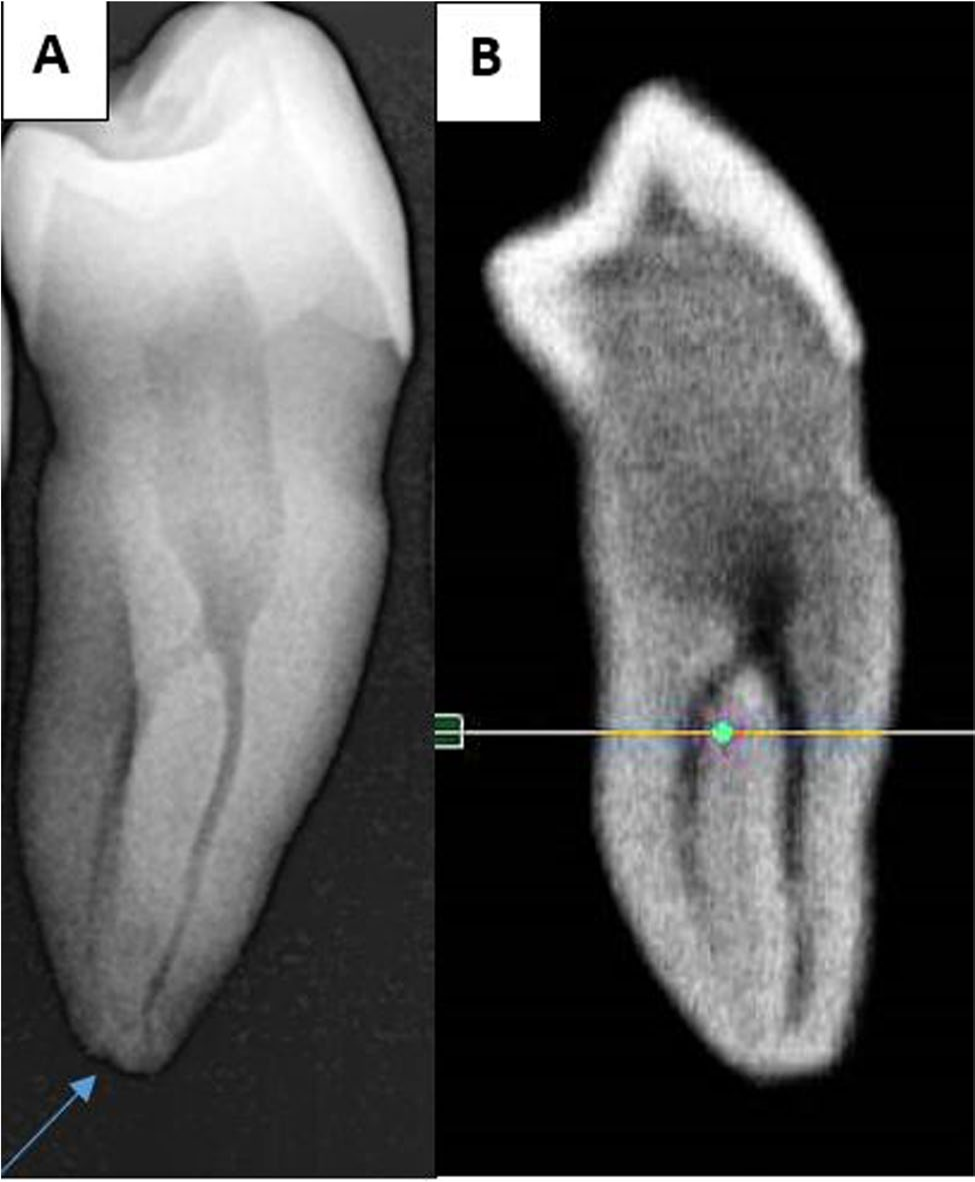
Another Vertucci class VII or Ahmed ^1^TN^1–2−1–2^. A: captured using digital sensor imaging B: one of the CBCT slices. The arrow highlights the small additional canal, which altered the classification and was detected in the digital sensor image but was not identified in the CBCT sections
